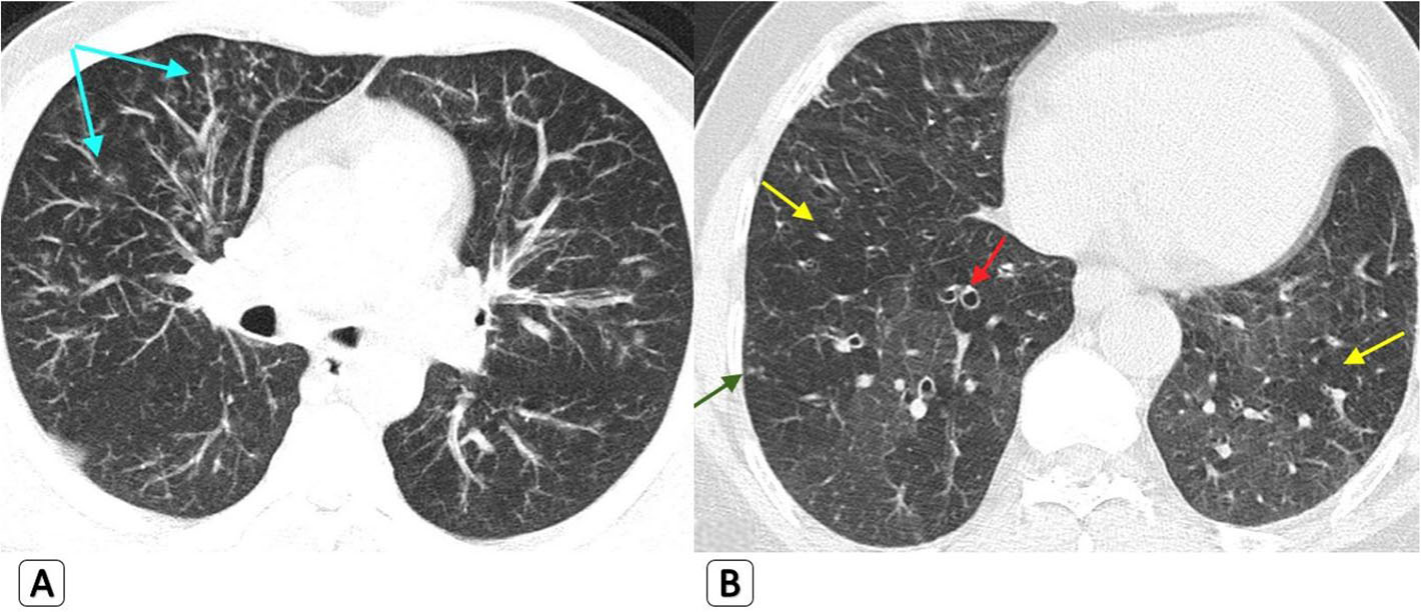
Mixed parenchymal/alveolar and airway abnormalities seen with H1N1: 47-year-old male H1N1 patient. a Axial HRCT chest lung window with “MIP” reconstruction demonstrating tree in bud nodules (blue arrows) and small ground-glass opacities. b Axial HRCT lung window showing mild bronchiectatic changes with “signet ring sign” (red arrows), patchy areas of mild air trapping (yellow arrows), and tiny few right lower sub-pleural tree in bud nodules (green arrow)
